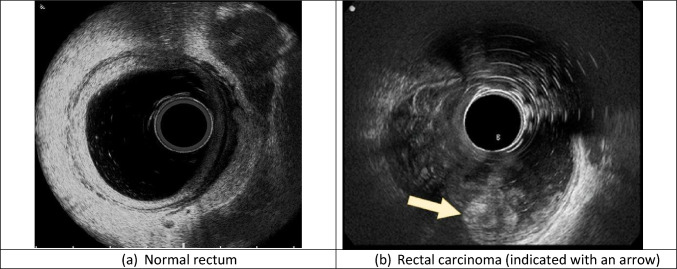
Endoanal ultrasound images of a water-filled rectum
